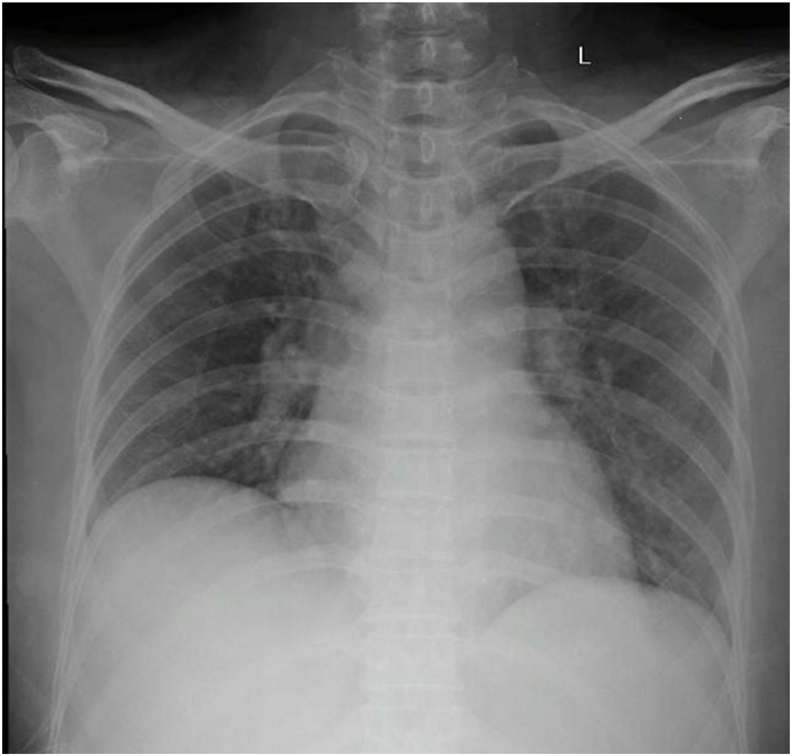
Chest X-ray showed bilateral pneumonia.
